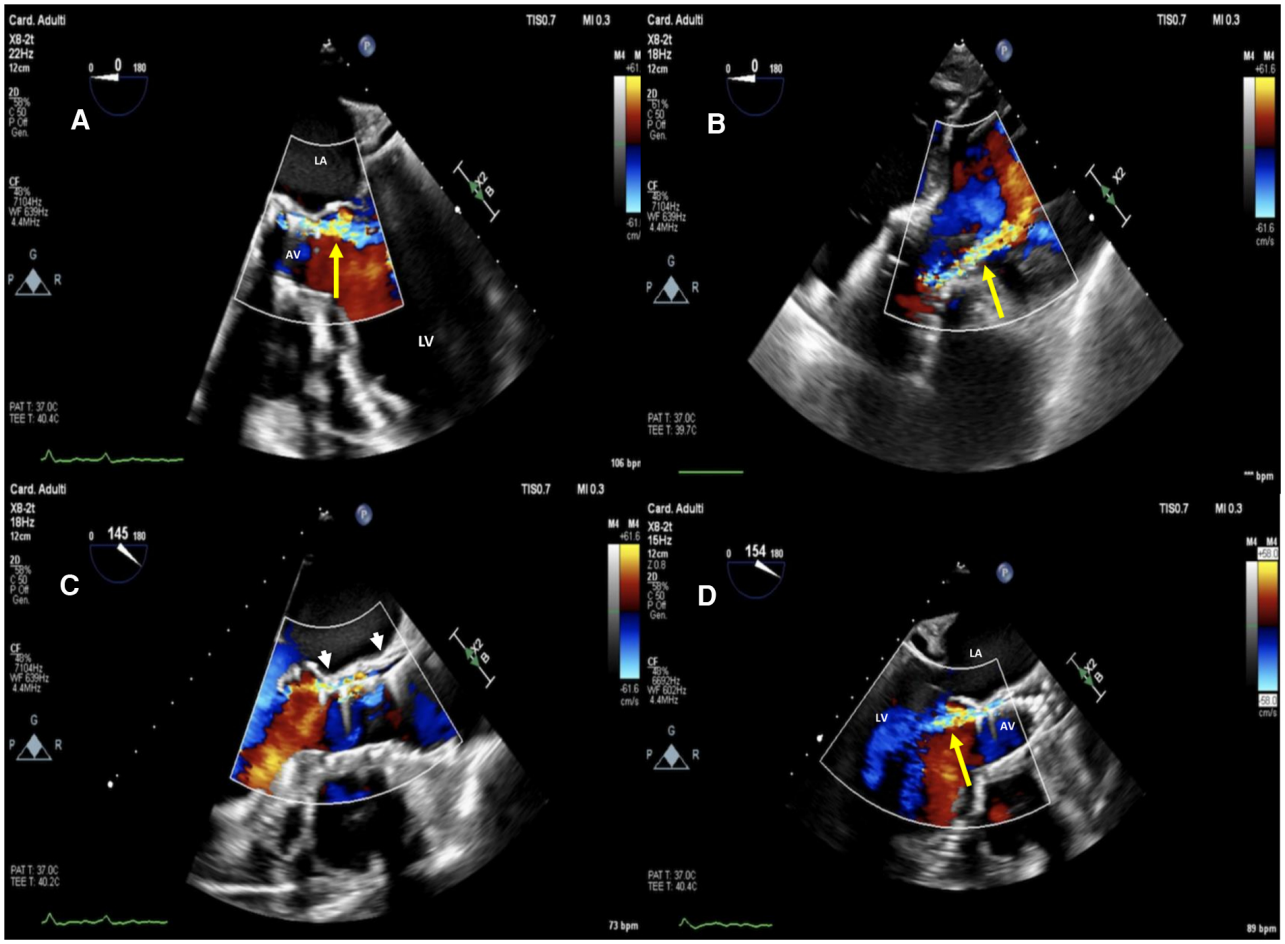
Baseline two-dimensional (2D) transesophageal echocardiography (TEE) color Doppler at mid-esophageal 5 chamber view (A), at deep transgastric 5 chamber view (B) and mid-esophageal AV long-axis view (C,D) showing a 26 mm SE evolut™ R® with moderate to severe regurgitation (yellow arrow) through a long, tortuous paravalvular leakage below the left coronary sinus (white arrowheads). La, left atrium; LV, left ventricle; AV, aortic valve.
